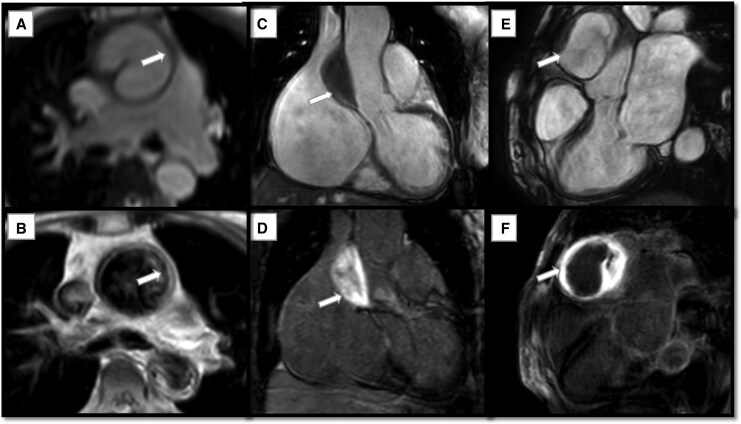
(*A* and *B*) Axial cut SSFP image showing thickened aortic wall (*A*). Axial cut T2-weighted image at the same level showing high signal intensity of the aortic wall (*B*). (*C* and *D*) Coronal cut SSFP image showing the mass encasing the ascending aorta extending from the sinotubular junction to just below the proximal aortic arch (*C*). Coronal cut late gadolinium enhancement image showing avid enhancement of the mass (*D*). (*E* and *F*) Three-chamber view SSFP image showing circumferential mass encasing the ascending aorta (*E*). Three-chamber view late gadolinium enhancement image showing avid enhancement of the mass with mid-myocardial basal anteroseptal hyperenhancement (*F*).
